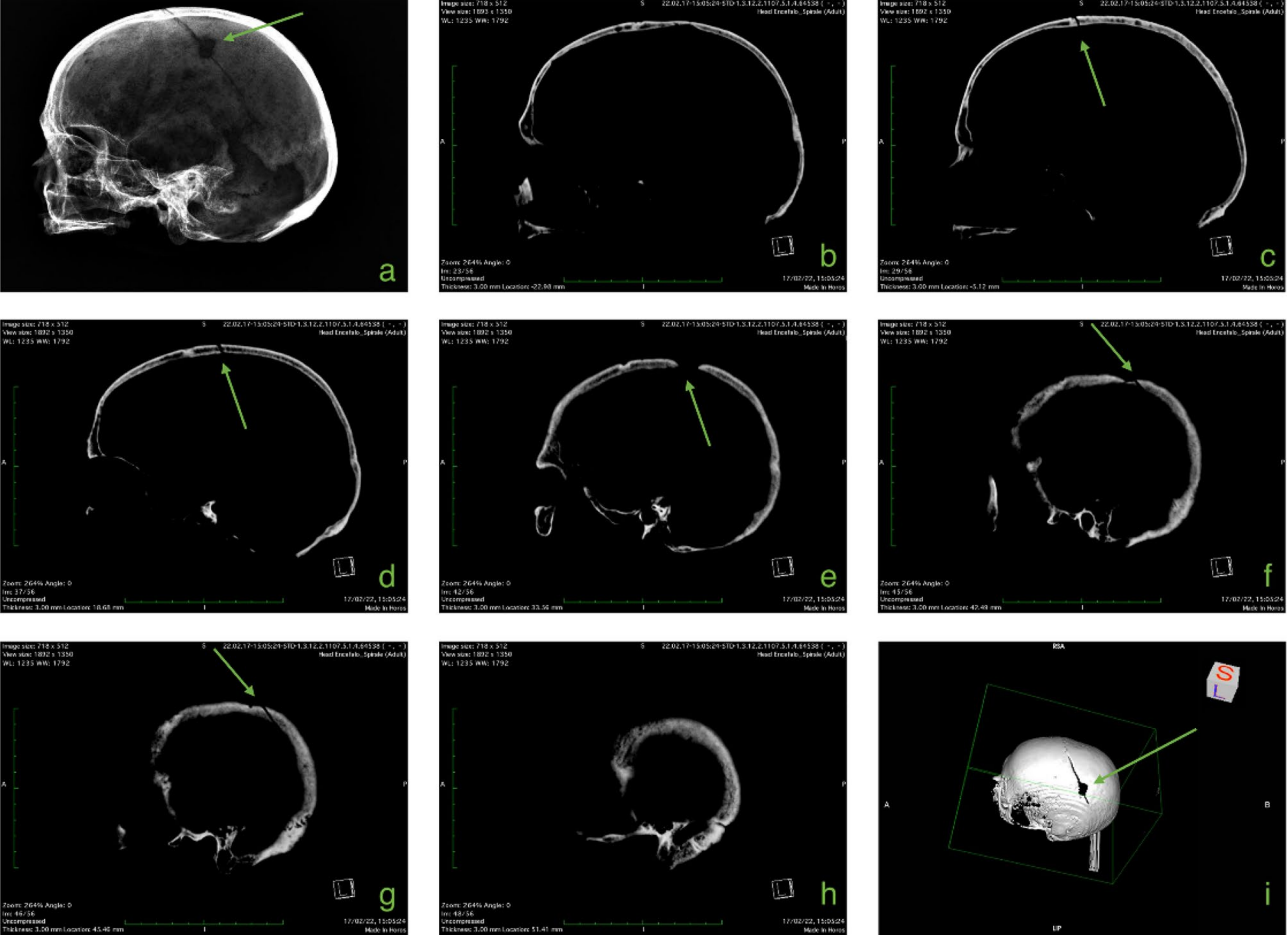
Radiological imaging of MI CG 21 O-US 4-1003. From (a–h), left lateral view of cranium. (a) X-rays imaging (green arrow: ante-mortem trauma). (b) CT image without signs of antemortem trauma. From (c–g), CT images of antemortem trauma probably followed by trepanation on left parietal bone. (h) CT image without signs of antemortem trauma. (i) Three-dimensional representation of cranium from superior lateral view of the left side.
